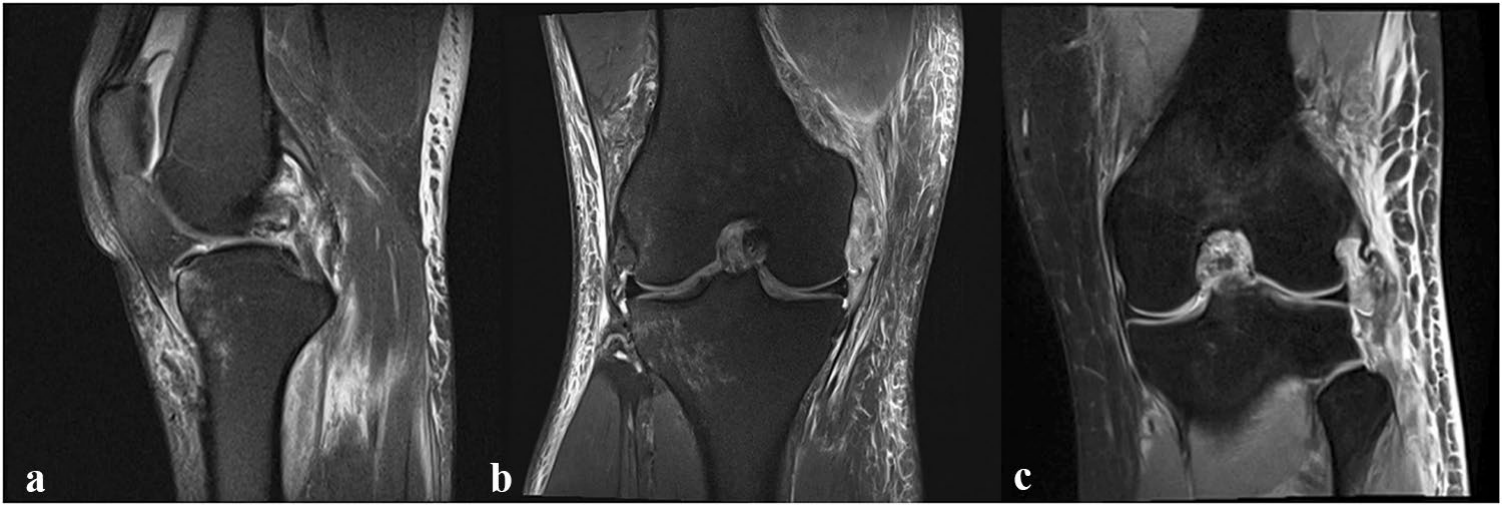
Preoperative MRI of a combined PCL lesion with proof of intraligamentous PCL tear (a) and either PMC injury with femoral MCL avulsion (b) or PLC injury with fibular LCL avulsion (c). *PCL* posterior cruciate ligament; *PMC* posteromedial corner; *PLC* posterolateral corner. *MCL* medial collateral ligament. *LCL* lateral collateral ligament
